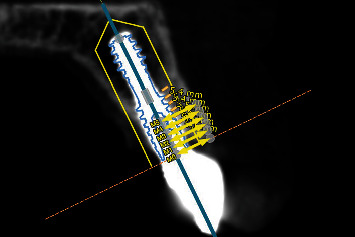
BBT measurement at the upper 5 mm of the implant on a CBCT scan at T12.
